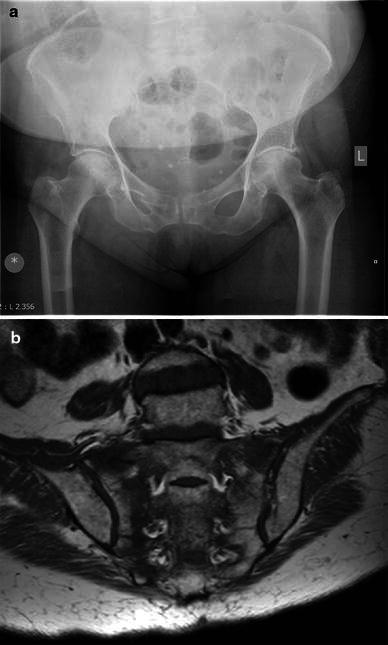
a Anteroposterior radiograph of pelvis of an 84-year-old woman with back pain showing right superior and inferior pubic rami fractures. b Coronal T1-weighted magnetic resonance image scan of same patient showing sacral osteoporotic fracture
